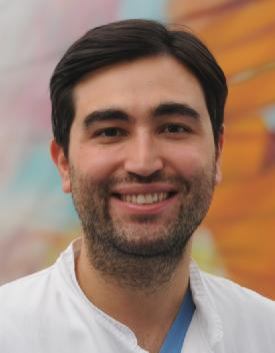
Burak Oezdemir, MD, is a cardiologist at the Department of Cardiology and Angiology, University Hospital Bergmannsheil, Ruhr University Bochum, Germany. He completed his specialist training in internal medicine and cardiology in 2024 and has a strong clinical focus on multimodal cardiac imaging, structural heart disease, and acute cardiovascular care. Dr. Oezdemir is actively involved in case-based teaching and research, with particular interest in echocardiography, HCM, and pathophysiological insights into rare cardiac variants. He is also engaged in interdisciplinary case conferences and supports advanced clinical education initiatives within his department.
